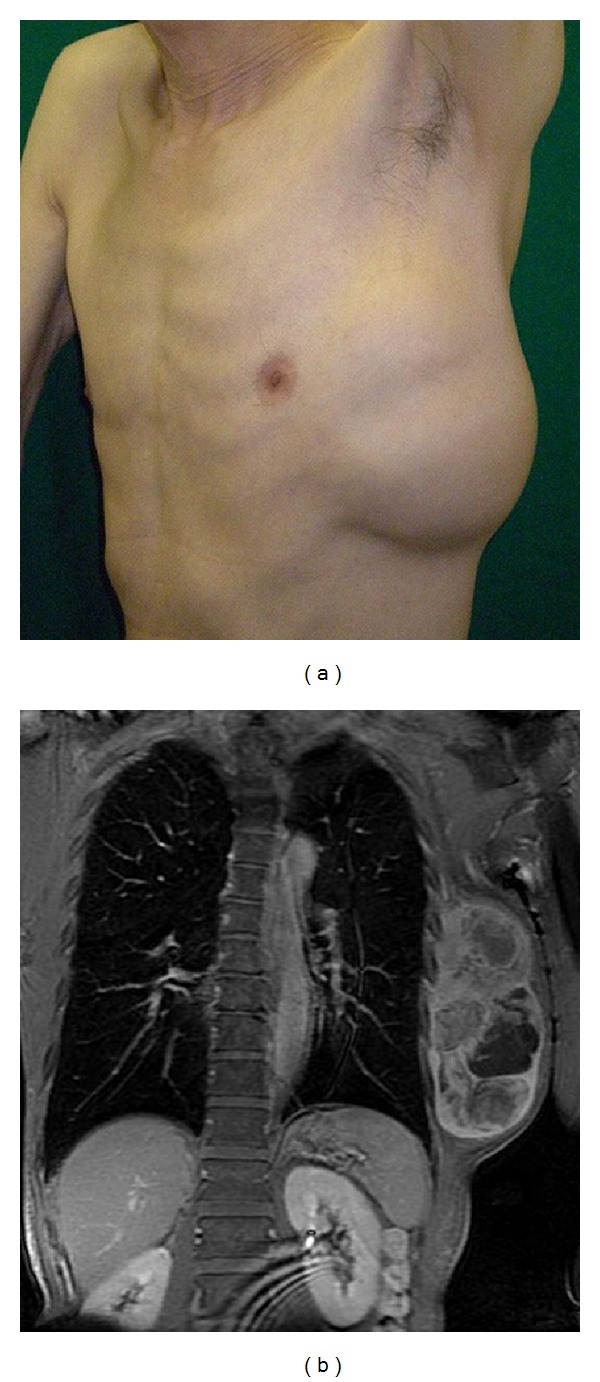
(a) Tumor of left lateral chest wall. (b) Preoperative magnetic resonance image of the tumor.
